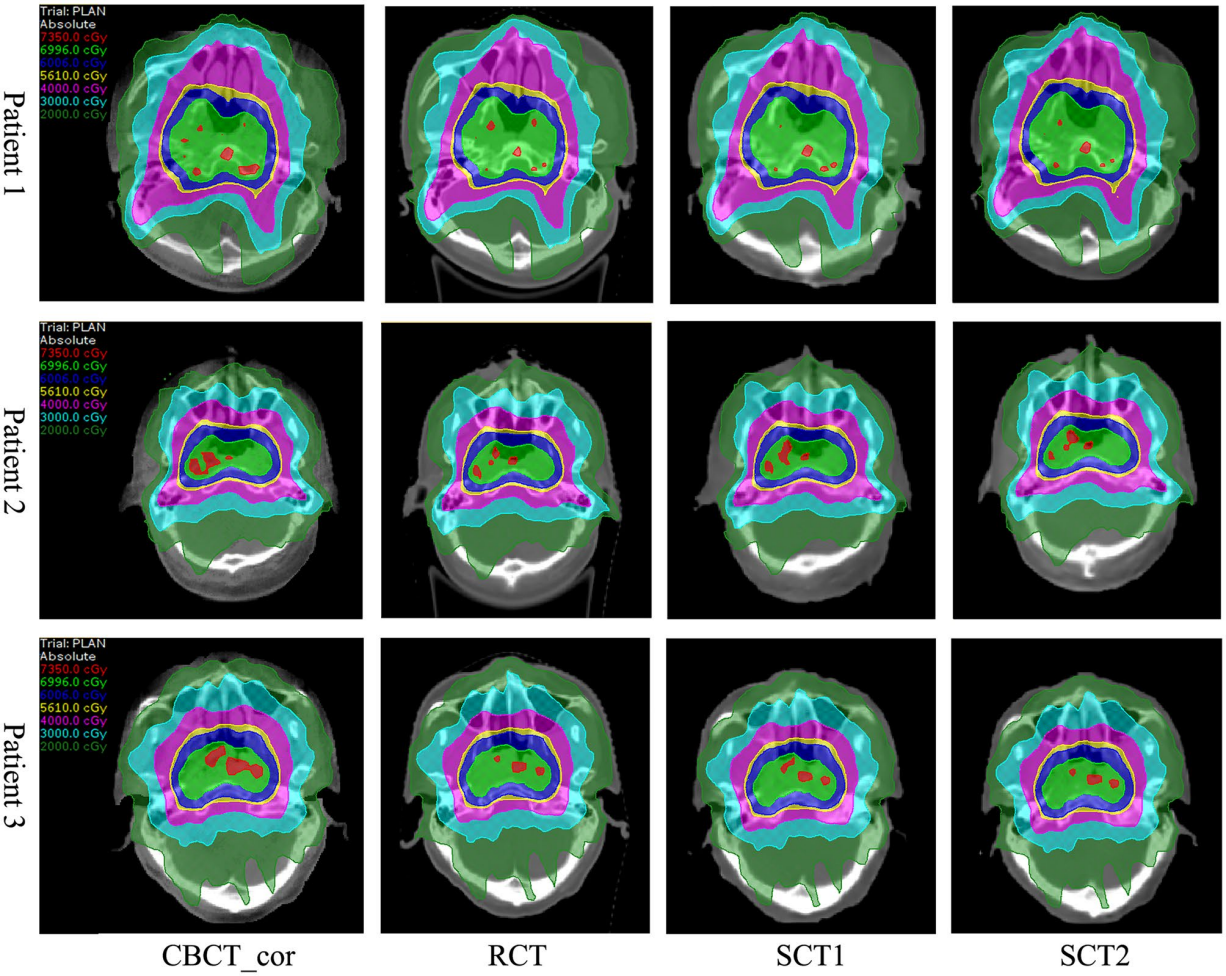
The dose distributions for three validation patients on CBCT_cor, RCT, SCT1 and SCT2 were displayed.
